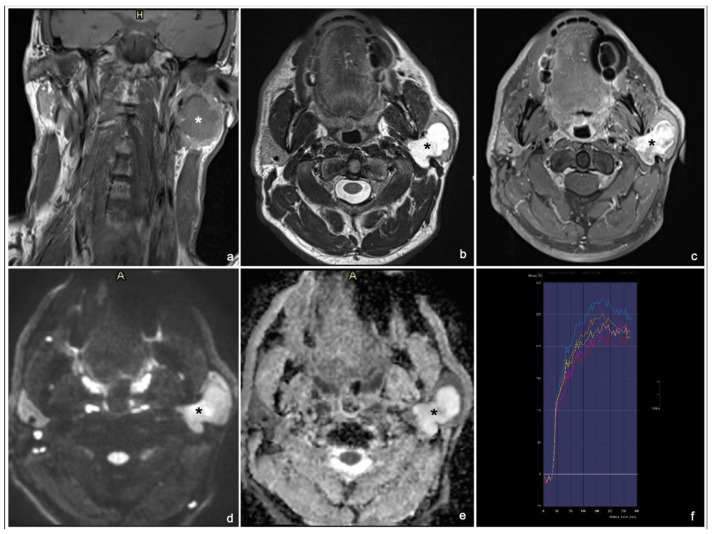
54 years old man with a pleomorphic adenoma of the left parotid gland: coronal T1 TSE (a), axial T2 TSE (b), late T1 TSE FS + Gd (c), DWI and ADC map (d,e) and DCE signal intensity-time S(t)-curve type A (f). * tumor. The lesion has an inhomogeneous, intense enhancement after contrast agent injection (normalized late T1 + Gd SI ≥ 2) (c) and a high signal on T2 (normalized T2 SI ≥ 3.5) (b).
